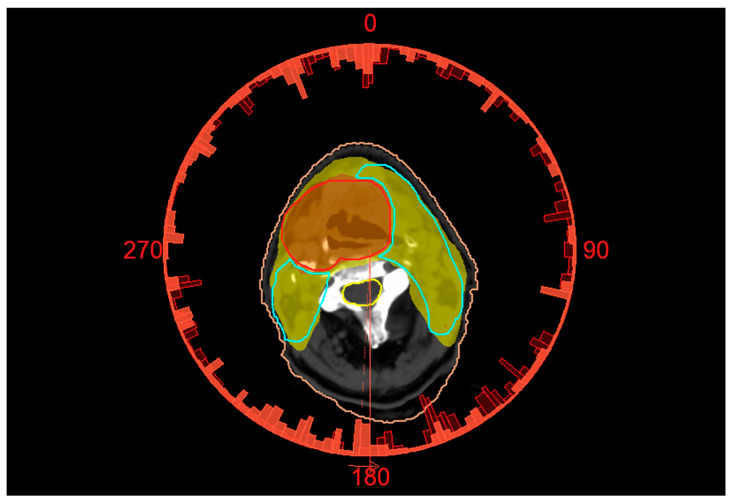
Computed tomography image with contoured structures: PTV1 (red); PTV2 (green) and spinal cord (yellow). Two full arcs of VMAT treatment plan with dose distribution around the two PTVs. The presented isodose areas of orange and yellow correspond to 95% of the prescribed doses of PTV1 and PTV2, respectively.
